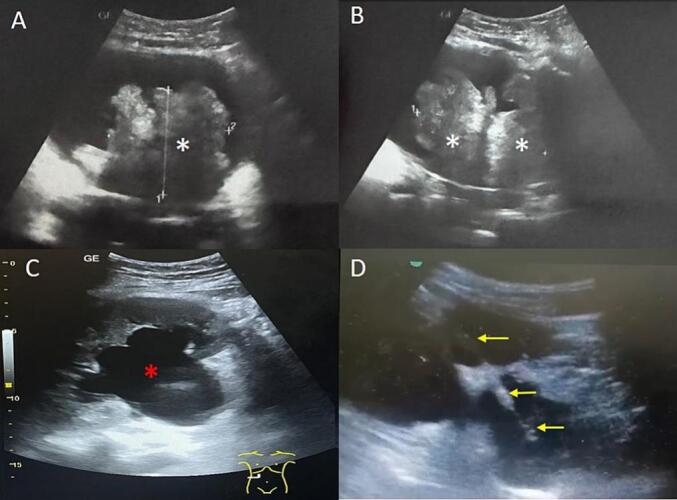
Renovesical ultrasound guiding nephrostomy placement: (A, B) Bladder lesion (white asterisk); (C) Pyelocaliceal dilation (red asterisk); (D) Puncture needle trajectory (yellow arrow). (For interpretation of the references to colour in this figure legend, the reader is referred to the web version of this article.)
